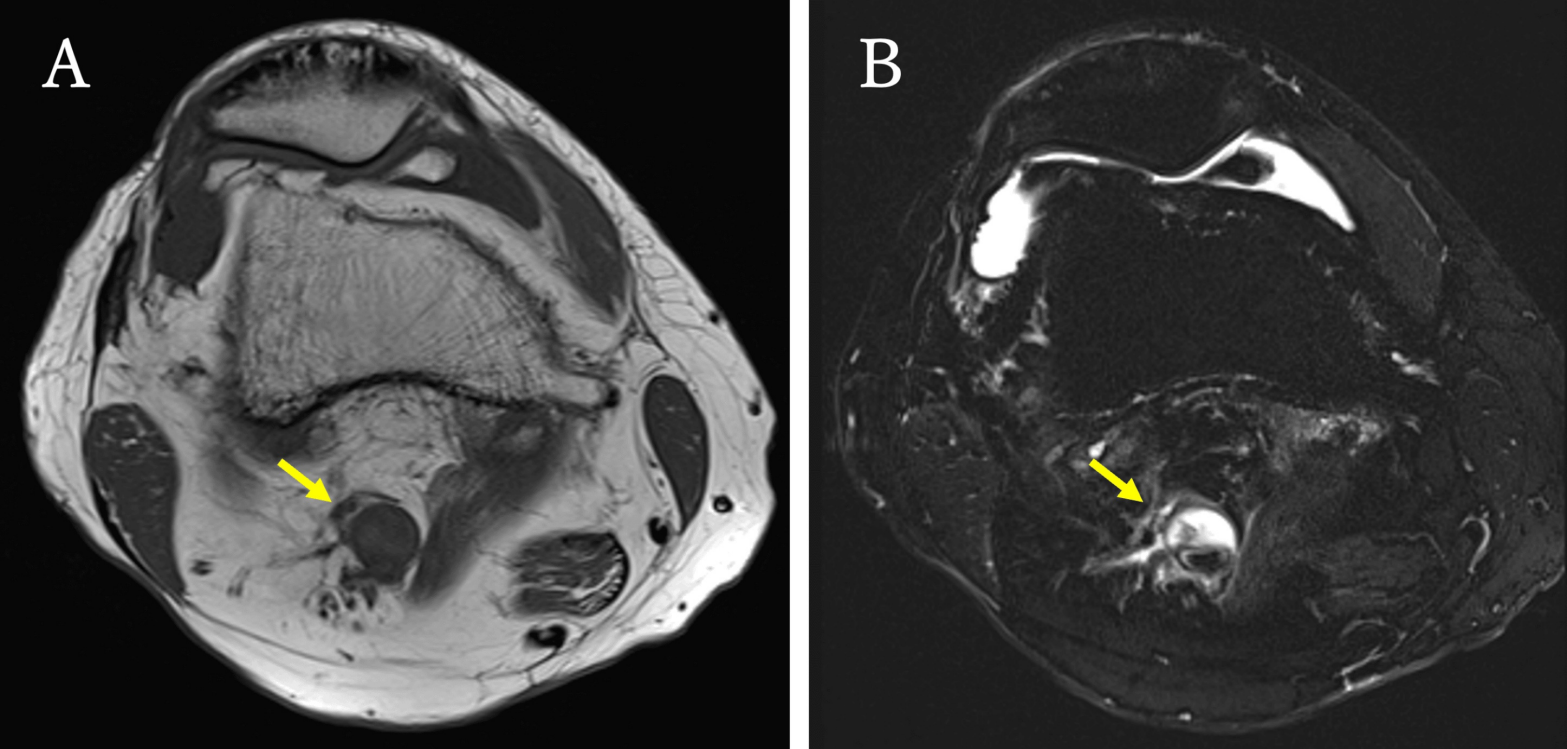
Magnetic resonance imaging. (A) T1-weighted imaging shows a low-intensity area in the right popliteal artery (arrow). (B) T2-weighted imaging shows a high-intensity area in the same region of the right popliteal artery (arrow).
